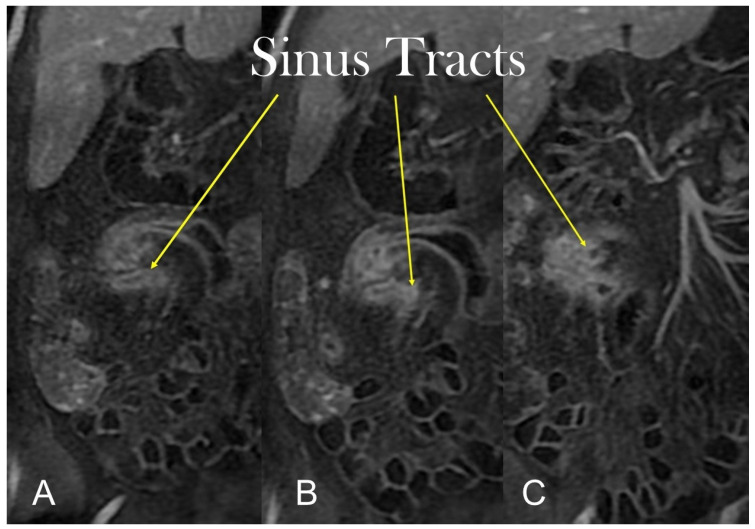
Coronal (A–C) contrast-enhanced fat-suppressed T1-weighted images of the same patient show the sinus tracts that represent a sealed-off perforation that develops into a phlegmon or, if infected, into an abscess (9) but does not reach adjacent organs or the skin. Therefore, they end up blind.
